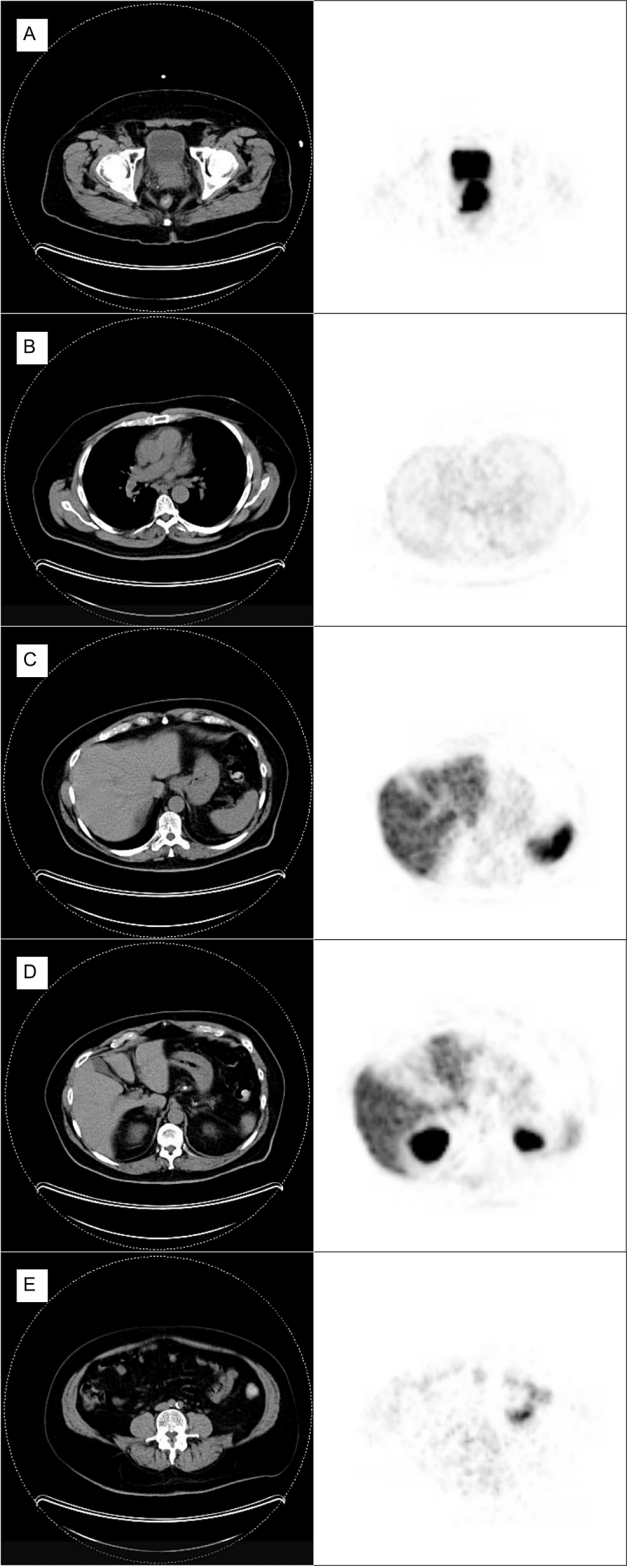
PSMA-PET/CT representative images (A) Prostate shows an abnormal expression of PSMA consistent with PCa. No evidence of pelvic lymph node metastasis was found. (B) Mediastinum shows no abnormal expression of PSMA and no evidence of pulmonary lymph node metastasis. (C) The liver shows no abnormal expression of PSMA. Imaging is consistent with the liver’s typical metabolic activity. (D) Adrenal glands show increased metabolic activity consistent with their typical activity. The patient also has nephrolithiasis, which may have contributed to the imaging observed. (E) Retroperitoneal lymph nodes do not show evidence of increased metabolic activity. PSMA: Prostate-specific membrane antigen; PET/CT: Positron emission tomography/computed tomography; PCa: Prostate cancer.
